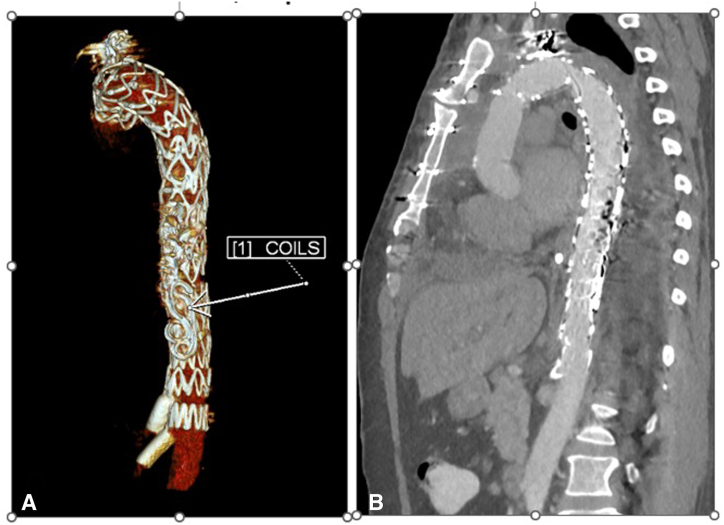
CT aortography post endoleak repair. 3D reconstructed image (A) shows the coils along the posterolateral aspect of the TEVAR stent used to occlude the endoleaks. There is extension of the stent inferiorly with stenting of the celiac axis and SMA as well. Sagittal oblique reformat (B) does not show any opacification of the false lumen, indicating successful management of the endoleaks. *CT*, Coronary angiography; *SMA*, superior mesenteric artery; *TEVAR*, thoracic endovascular aortic repair*.*
